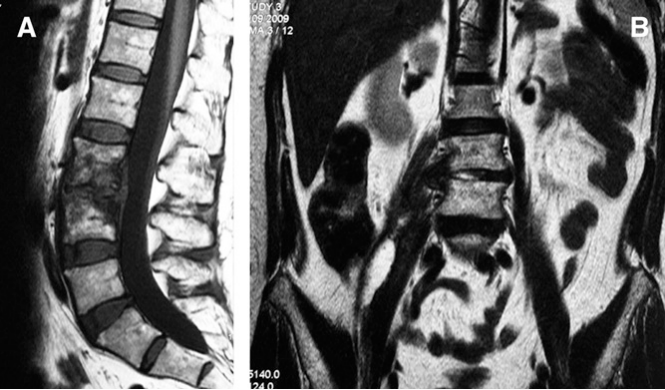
(A) MRI of the spine. Hypointensity on T1-weighted images at the L3-L4 level and narrowing of the L3-L4 intervertebral disc space. (B) Hyperintesity on T2-weighted images and marked widening of the right iliopsoas muscle.
